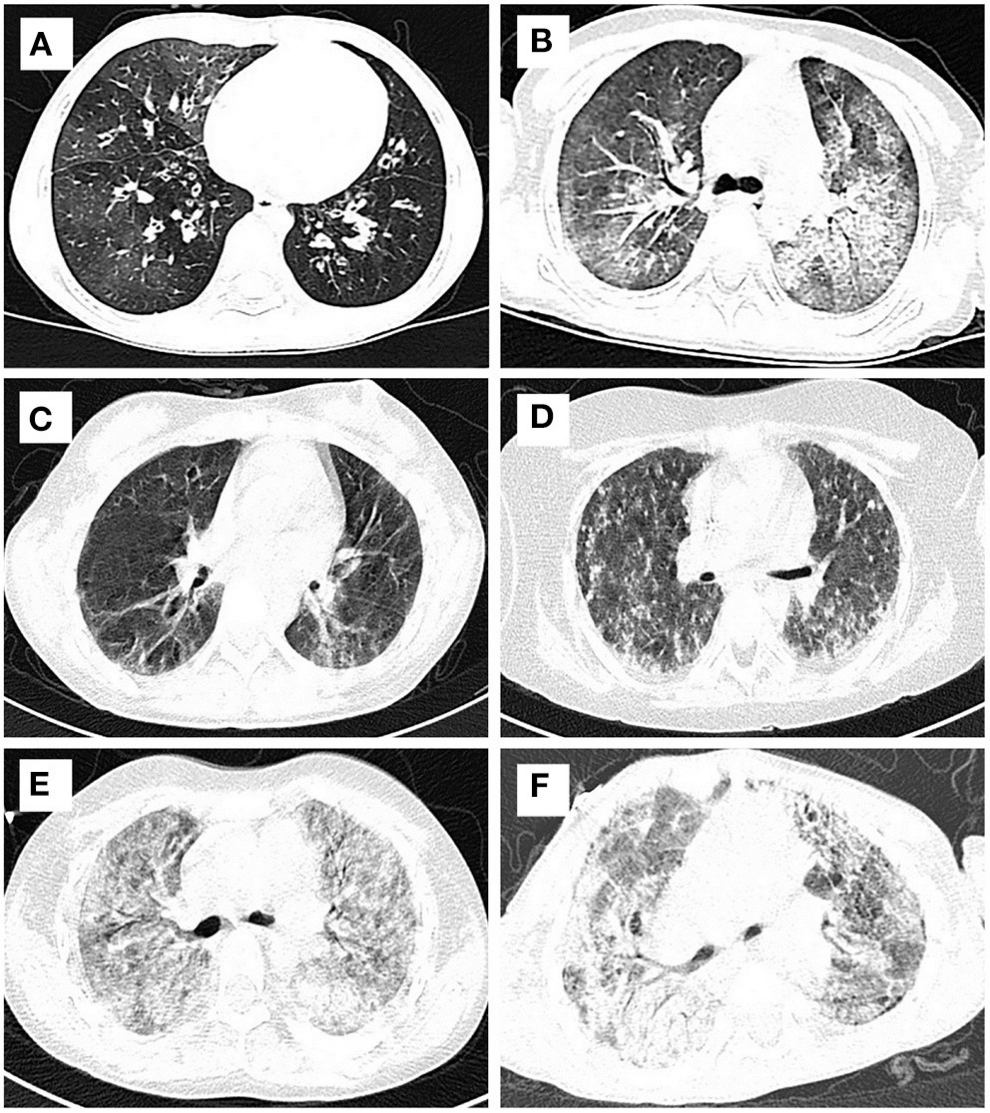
Typical HRCT imaging of patients with DRC. Mosaic perfusion, interlobular septal thickening and ground glass opacity from bronchiolitis obliterans (A) and alveolar proteinosis (B); reticular and linear opacities and pleural thickening from juvenile dermatomyositis (C) and pulmonaty fibrosis (D); ground glass opacity, interlobular septal thickening, and reticular & linear opacities from diffuse alveolar hemorrhage syndromes (E) and Sting associated with vasculopathy with onset in infancy (F).
